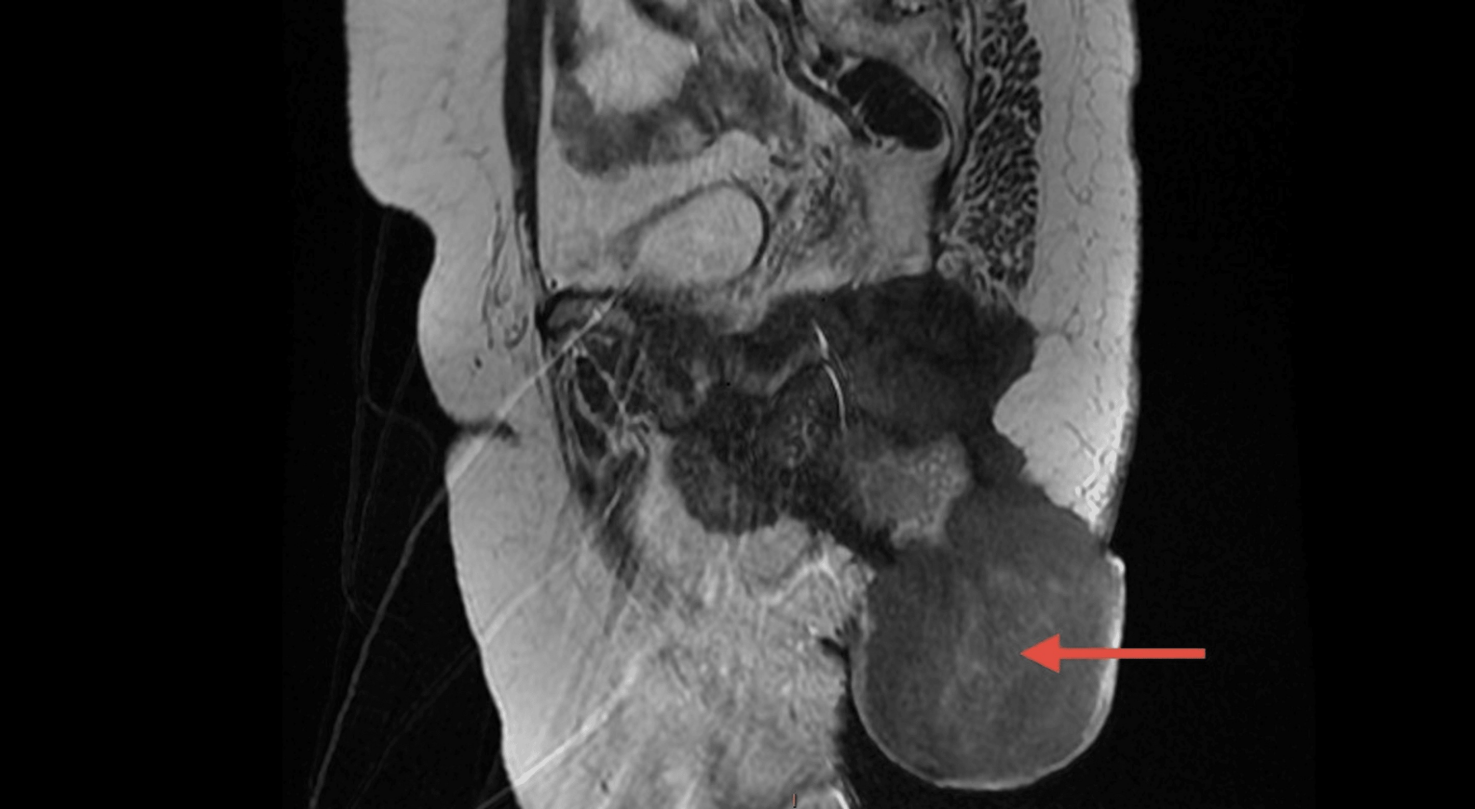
Sagittal section of a pelvic MRI Arrow points to the mass infiltrating the ischiopubic fossa with irregular contours, poorly defined, invading the levator ani muscle, measuring 70x62 mm.
